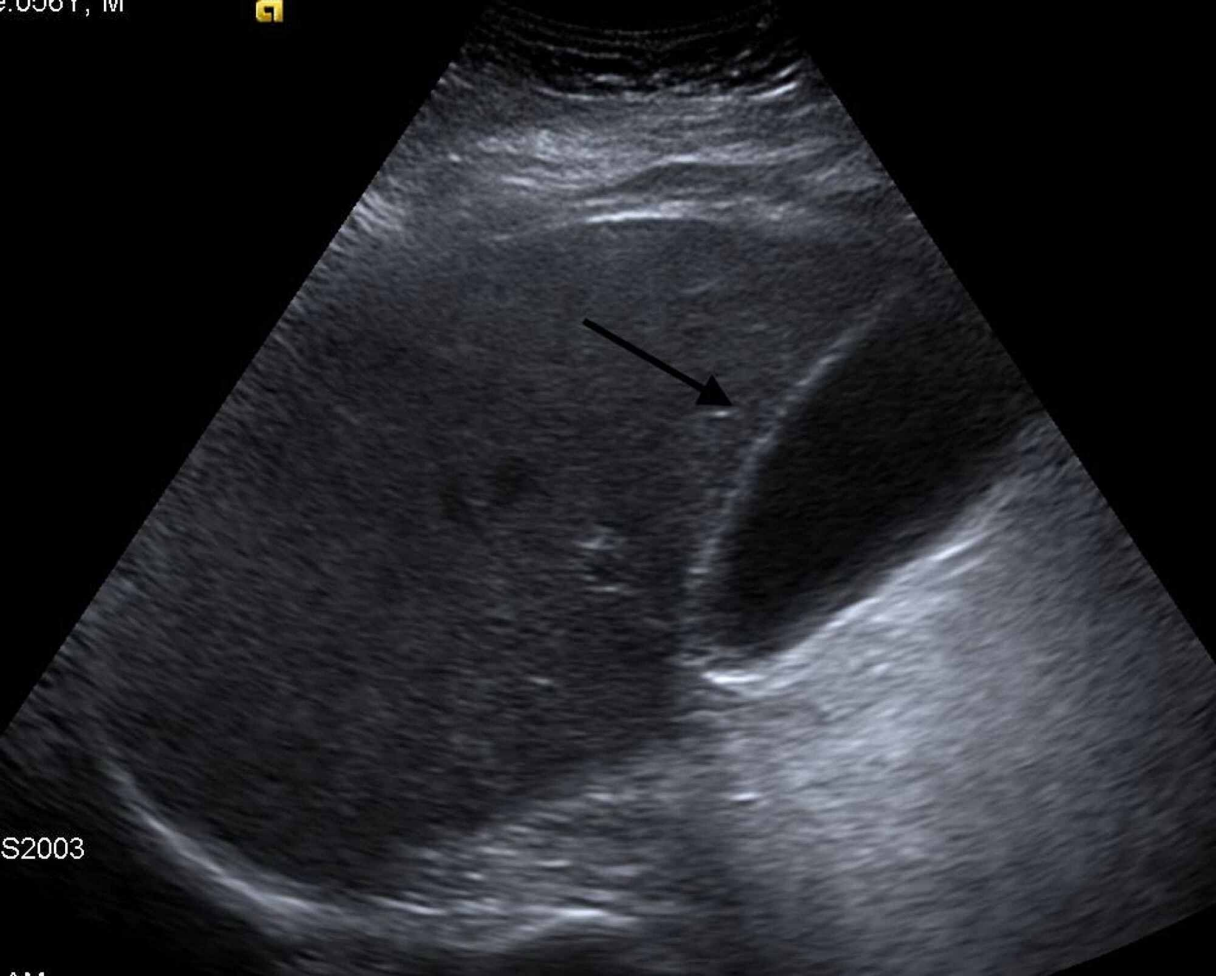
Gallbladder ultrasound shows no gallstones
